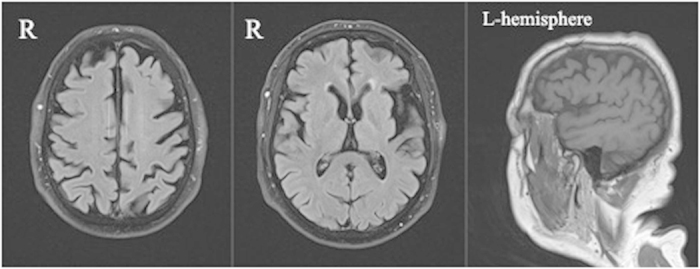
T1-weighted MRI of the brain of patient 9957.
